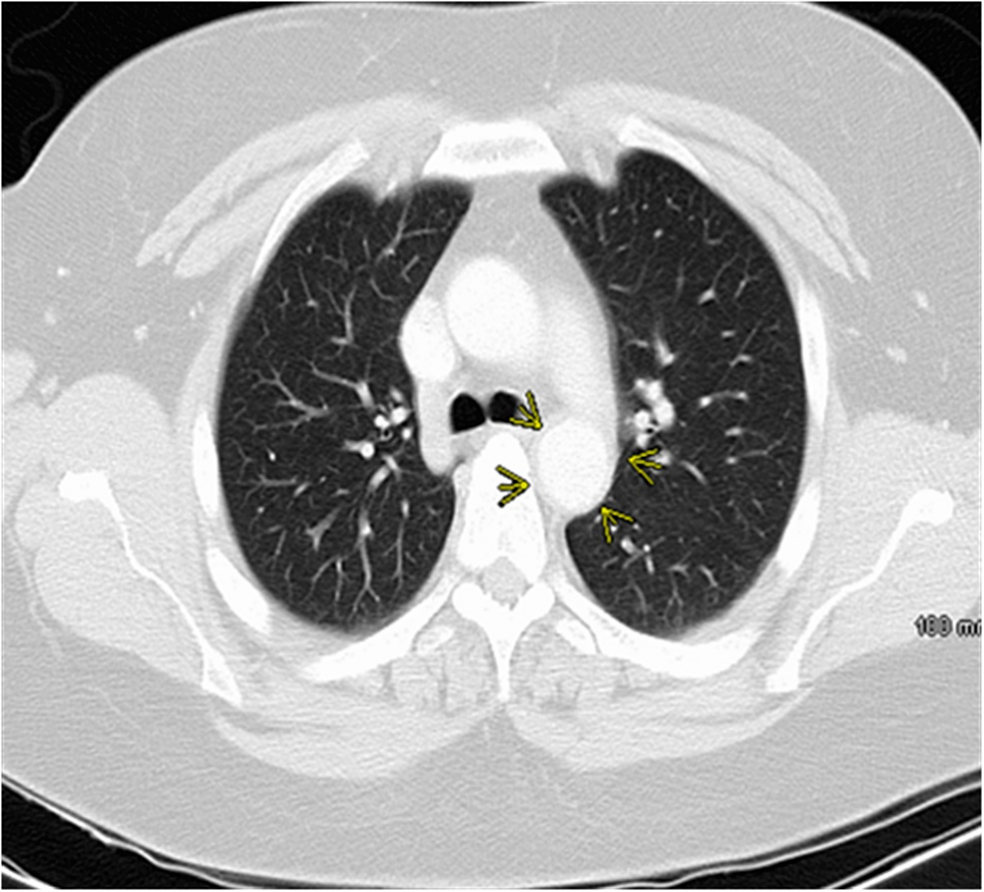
Computed tomography angiography of the chest, axial view The yellow arrow indicates concentric mural thickening of the descending segment of the abdominal aorta.
